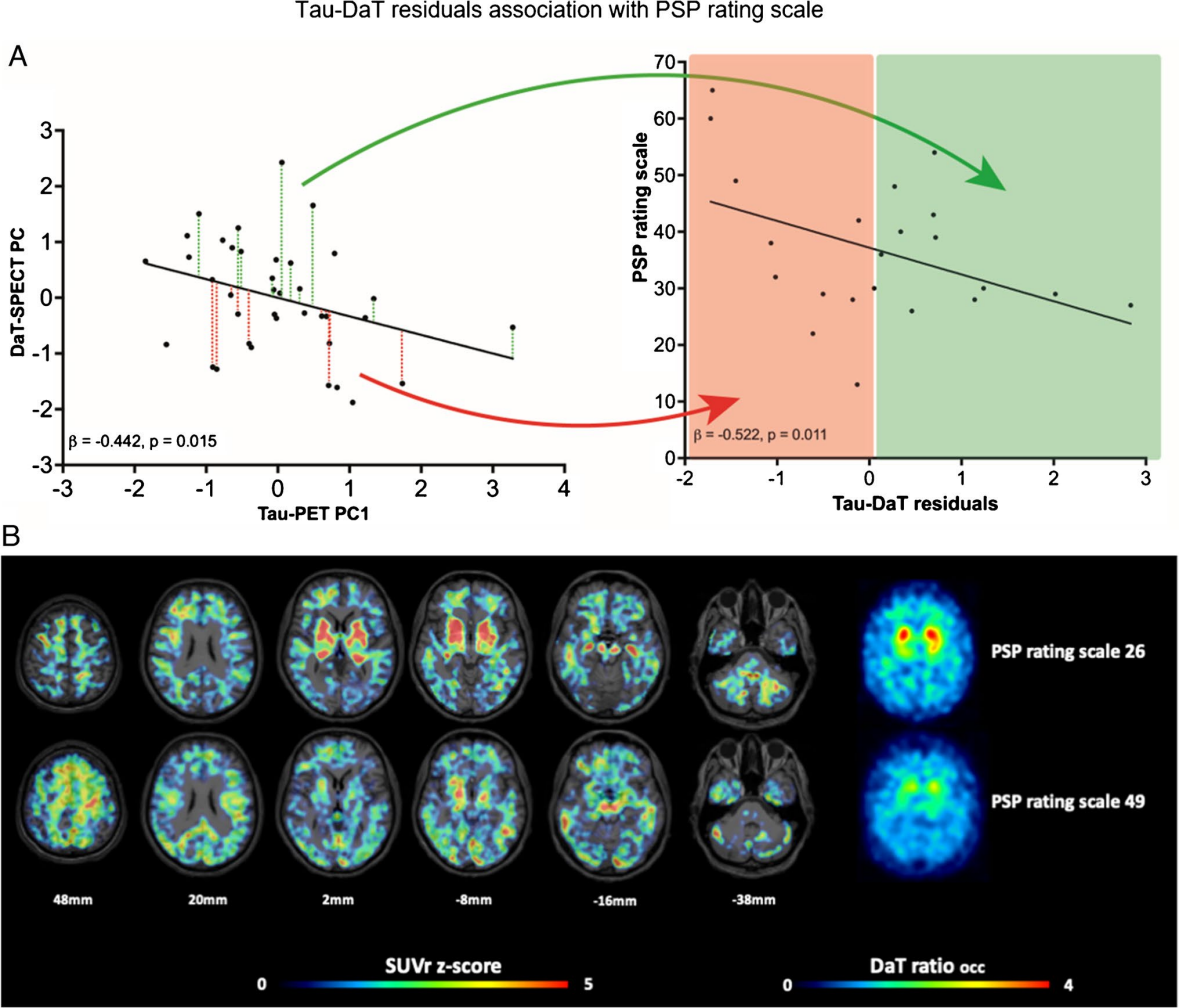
Preserved DaT availability relative to tau burden suggests concept of a motor reserve in patients with 4RT. A Residuals of the linear regression between the tau-PET principal component 1 (tau-PET PC1) and the DaT-SPECT principal component (DaT-SPECT PC) positive (green) and negative (red) were obtained as an index of preserved DaT availability despite tau burden. Preserved DaT availability was associated with lower disease severity in the PSP rating scale (right panel). Only patients with PSP rating scale scores are depicted. B Exemplary patients with 4R-tauopathies showing high tau burden, preserved DaT availability and mild clinical severity (upper row, 73y, female, PSP-CBS, PSP rating scale: 26) as well as moderate tau burden, strongly decreased DaT availability and severe clinical deterioration (lower row, 79y, female, PSP-CBS, PSP rating scale: 49). Axial slices show individual tau-PET z-scores on a standard MRI-template in contrast against healthy controls and individual DaT SPECT ratio images
